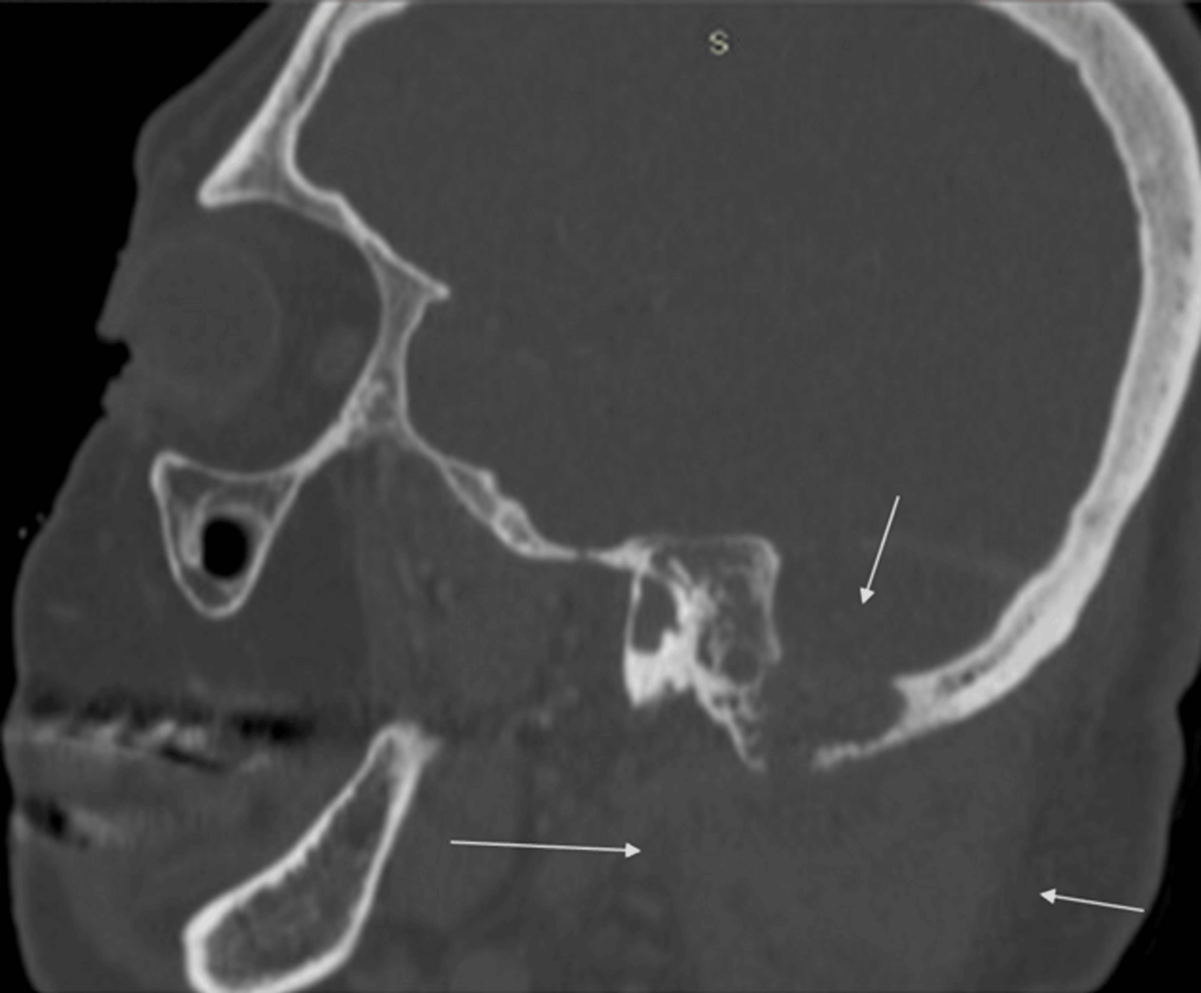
A sagittal plane of the CT scan of the temporal bone showing complete opacification of the right middle ear and the mastoid air cells, along with extensive erosion of the mastoid cortical bone and posterior cranial fossa along with the formation of the neck abscess (contained within the arrows).
